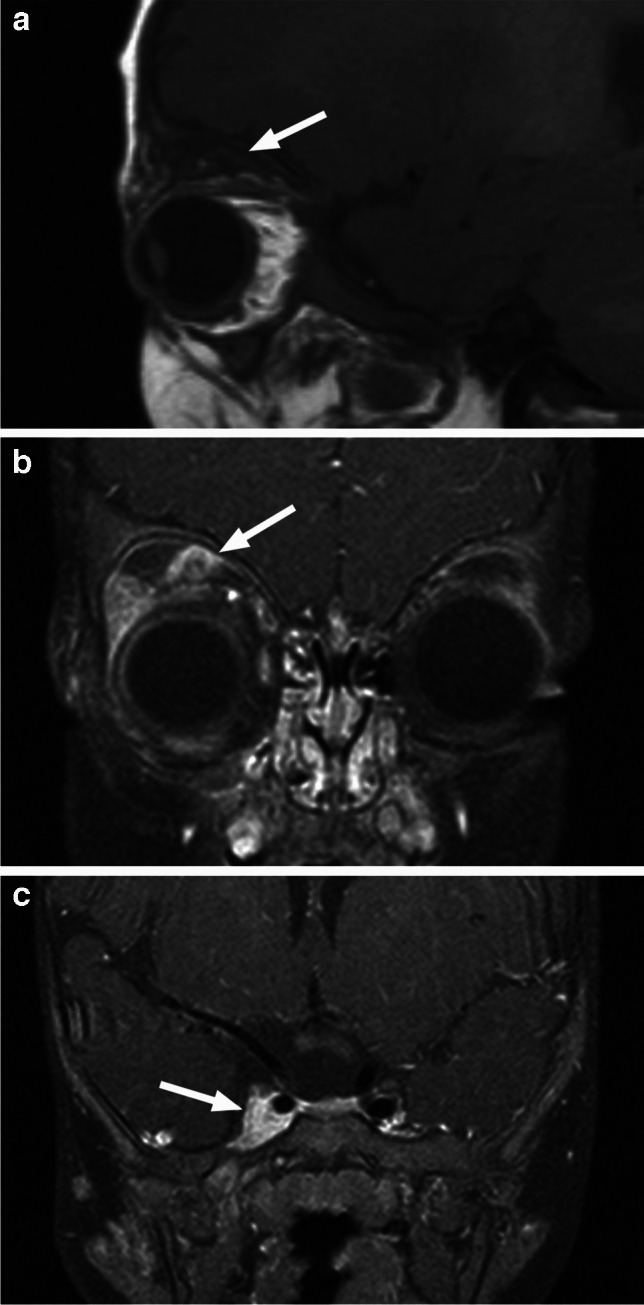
Plexiform neurofibroma. Sagittal T1-weighted image (a) shows a heterogenous lesion (arrow) in the superior extraconal compartment. There is heterogenous enhancement of the irregular mass (arrow) in the frontal nerve location and mild expansion of the orbit on postcontrast coronal T1-weighted image with fat saturation (b). A more posterior coronal image (c) shows extension of the lesion into the enlarged right cavernous sinus (arrow)
